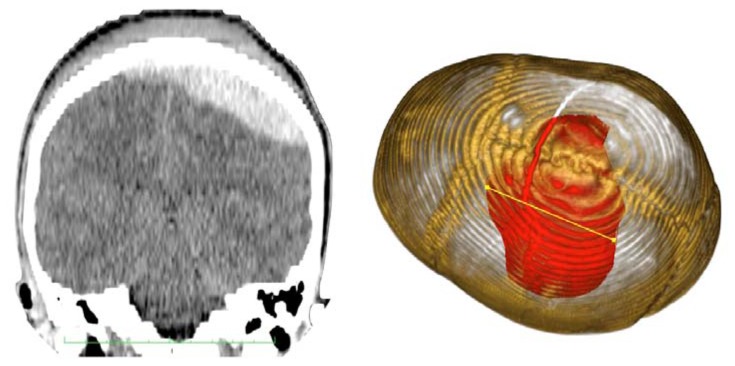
CASE 1. Preoperative CT scans. The visualization of the hematoma by transparency facilitates the spatial perception of the surgeon. In this particular case, the patient had an epidural hematoma beneath the superior sagittal sinus with a bilateral extension. The measured diameter is 7 cm (see also VIDEO 1).
